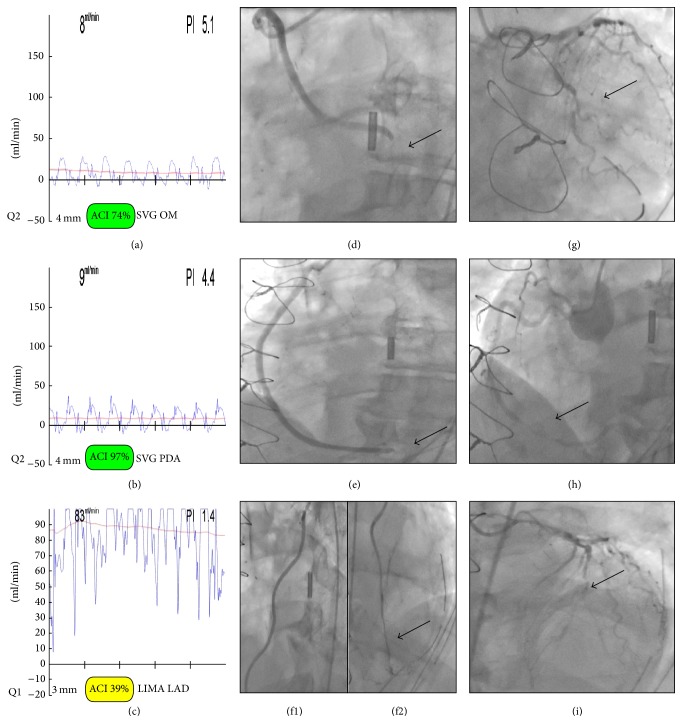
The VeriQ will display the Doppler spectrum at the default 5 seconds sweep rate as soon as a probe is connected, as shown in the figure. In Figures 1(a)–1(c) there is a flow-time curve of SVG to OM, SVG to PDA, and LIMA to LAD, respectively. After completion of all three grafts, there was good MGF in the arterial but not in the vein grafts. TTFM measurements with a mean arterial pressure of 80 mmHg were as follows: LIMA to LAD: MGF 83 ml/min and PI 1.4; LSV to OM: MGF 8 ml/min and PI 5.1; LSV to PDA: MGF 9 ml/min and PI 4.4 (Figures 1(a)–1(c)). An urgent angiography confirmed the patency of all three grafts with wide spread evidence of spasm to native coronary arteries (Figures 1(d)–1(i)). The arrows refer to spasm.
